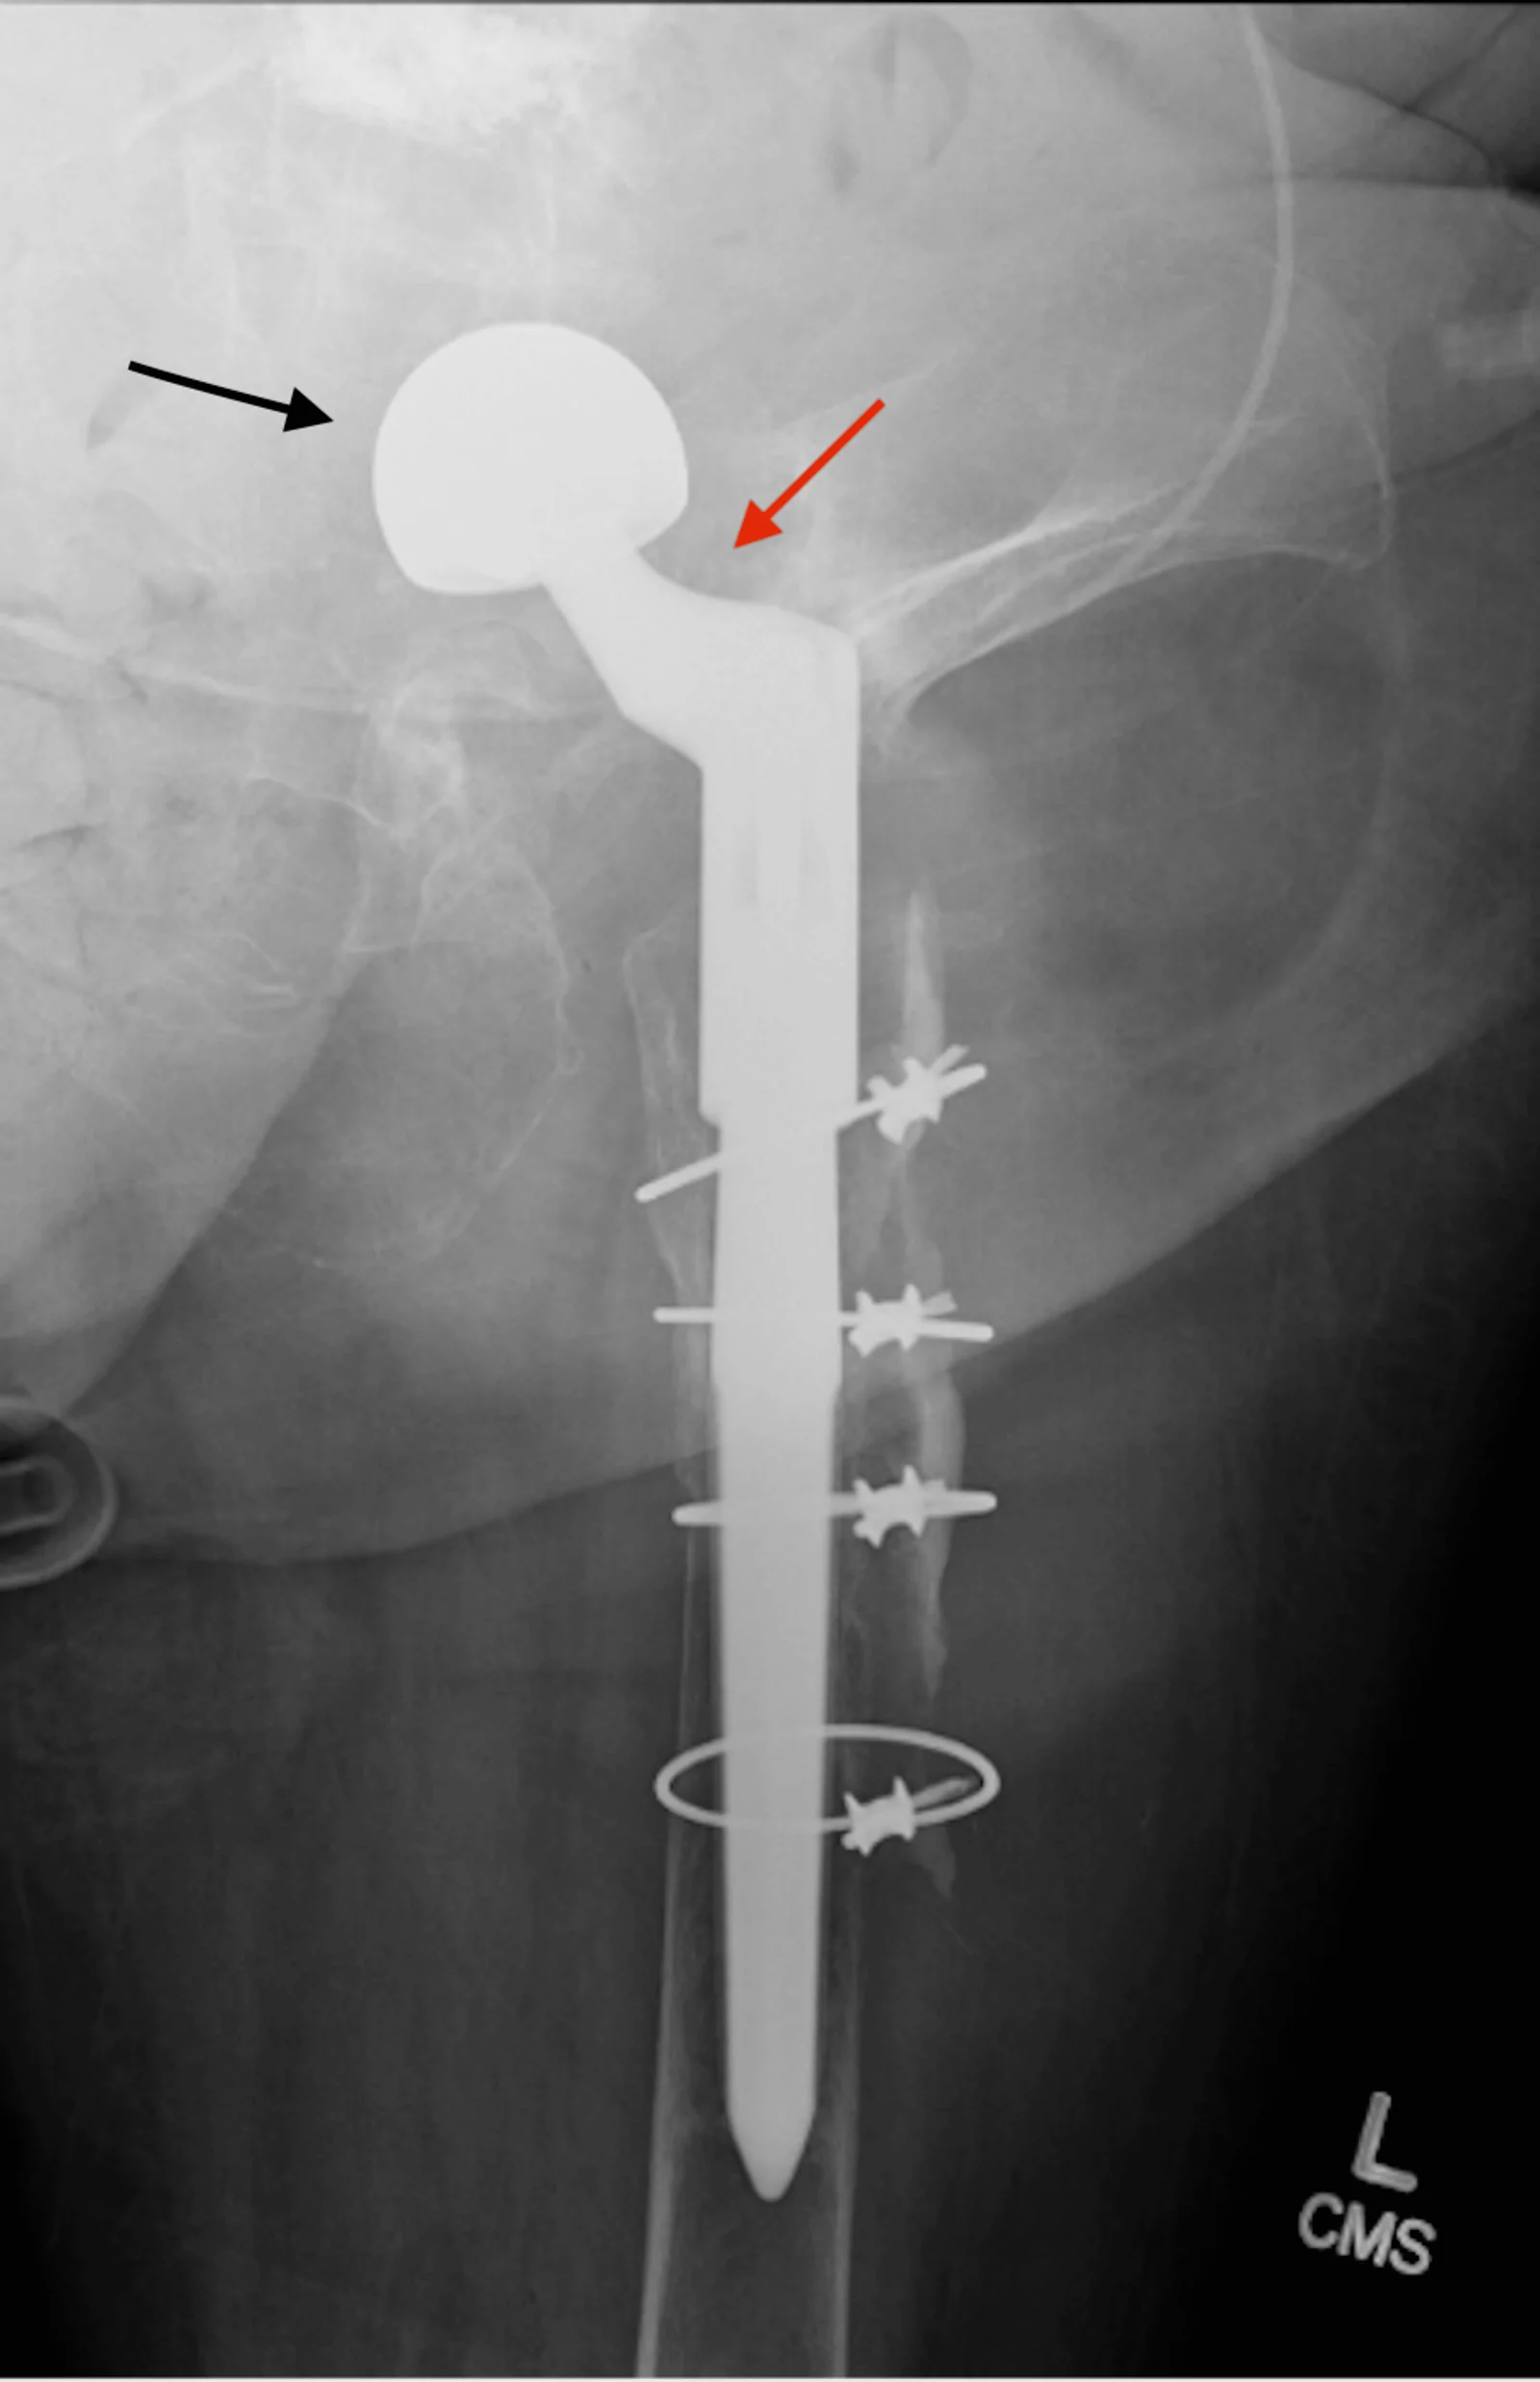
Anteroposterior (AP) radiograph of the left hip. The radiograph reveals periprosthetic lucencies with prosthetic femoral head (black arrow) and neck (red arrow) migrating superomedially through the acetabular wall.
